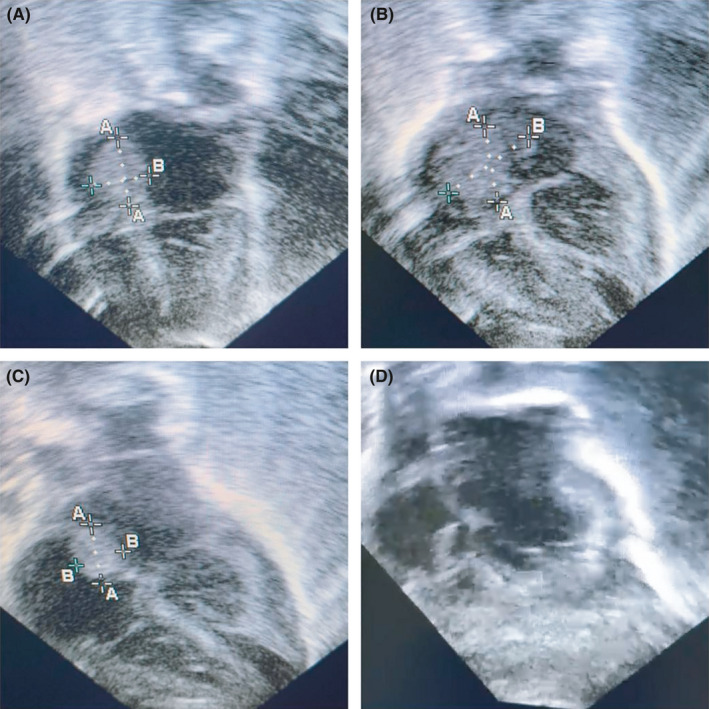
(A) Transthoracic ECHO revealed a large mass measuring 0.8 * 1 cm attached to the right interatrial septum and protruding into the left atrium. (B) A follow‐up ECHO after five days showed the progression of the vegetation size to 1.3 * 1.6 cm. (C) Significant regression in the size of the vegetation to 1.2 * 1 cm after adding rifampin. (D) Within 3 weeks of receiving combined antimicrobial therapy with rifampin, the vegetations were completely resolved without valvular dysfunction
